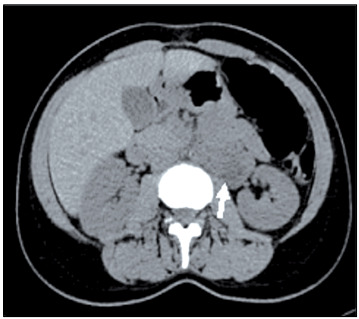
Axial abdominal computed tomography image showing hypodense mass lesion in the left anterior pararenal space with well-defined borders.
